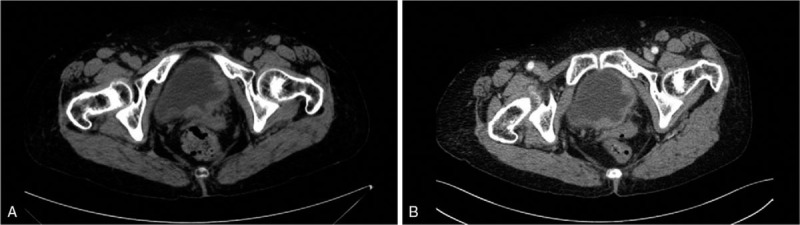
(A-B): Pelvic contrast-enhanced computed tomography (CT) showed multiple nodules in the bladder wall.
